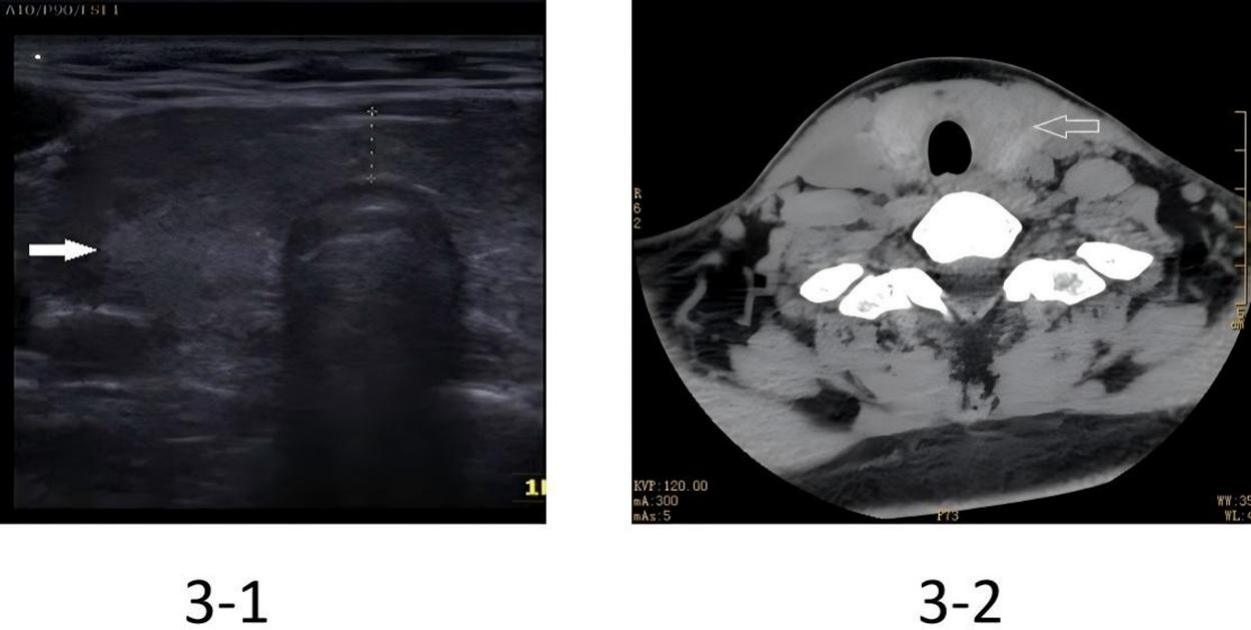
USG and CT scan revealed diffuse enlargement in the thyroid gland. Left: Thyroid ultrasound image; Right: Thyroid computed tomography image.
